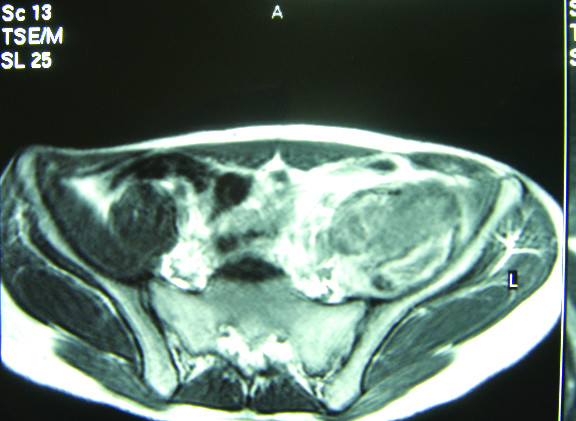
MRI scan. T1; Axial image, diffuse swelling is seen involving the left iliopsoas muscle showing heterogeneous signal intensity being iso-, hypo- and hyper-intense mostly due to late subacute haemorrhage. No associated retroperitoneal collection is seen.
